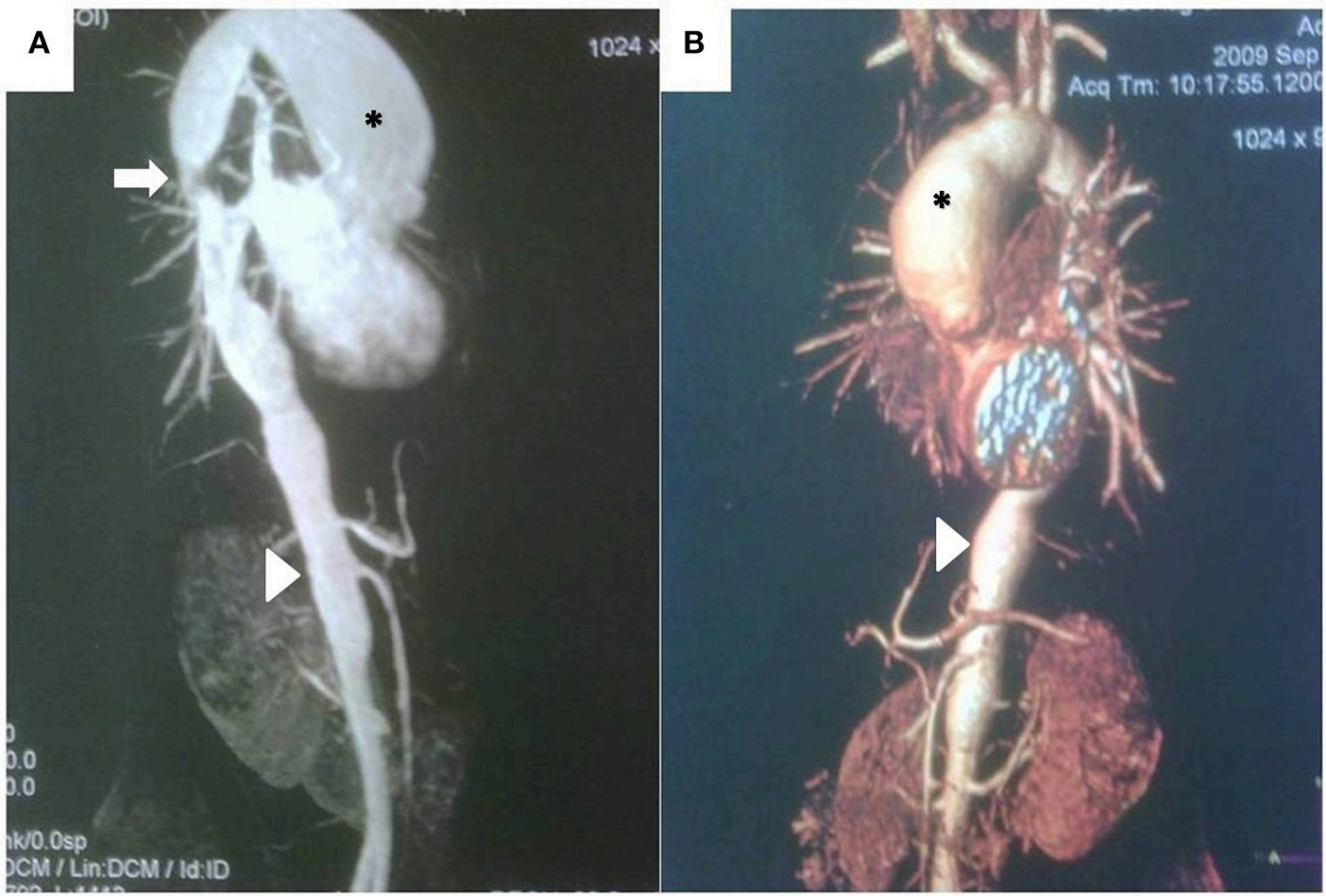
(A) Magnetic resonance angiography demonstrating large, secular aneurysm in the aortic arch (*), stenosis in the thoracic aorta (arrow), and irregularity of the thoracic and abdominal aorta, including stenotic areas and a long aneurysm (arrowhead) proximal to the renal arteries in a 13 year-old girl with recent-onset Takayasu arteritis. (B) CT scan and three-dimensional reconstruction of the same patient, demonstrating same findings, but providing better quality-anatomical details.
